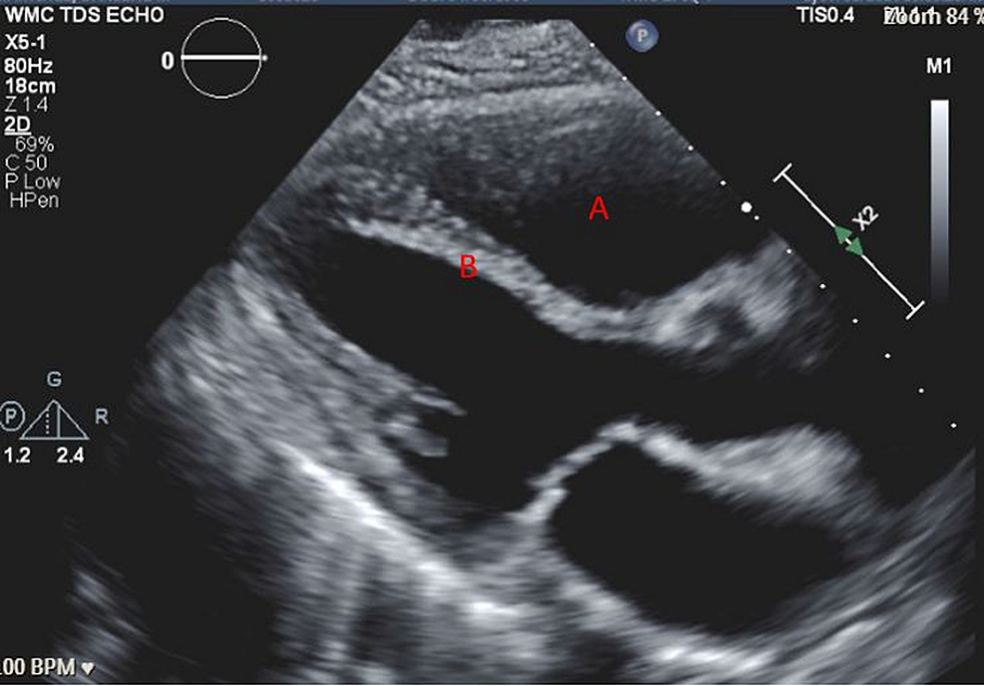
Preoperative TTE parasternal long axis view showing (A) dilated right ventricle and (B) flattened intraventricular septum as evidence of right-sided pressure and volume overload. TTE: transthoracic echocardiogram
